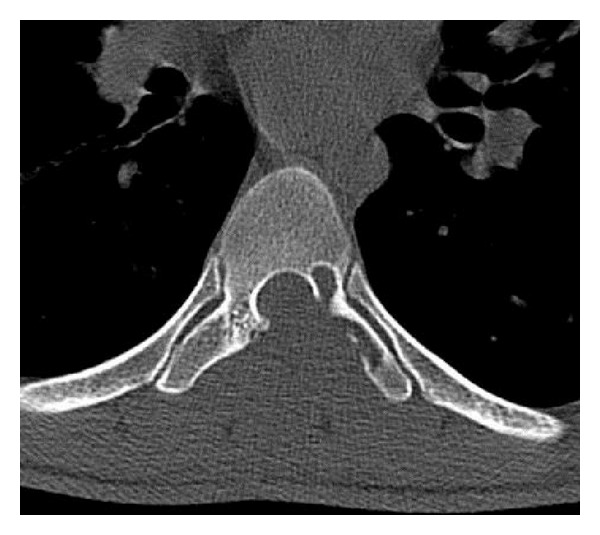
Axial CT scan showing soft tissue mass eroding through lamina and encroaching on thecal sac. There are no foci of calcifications.
